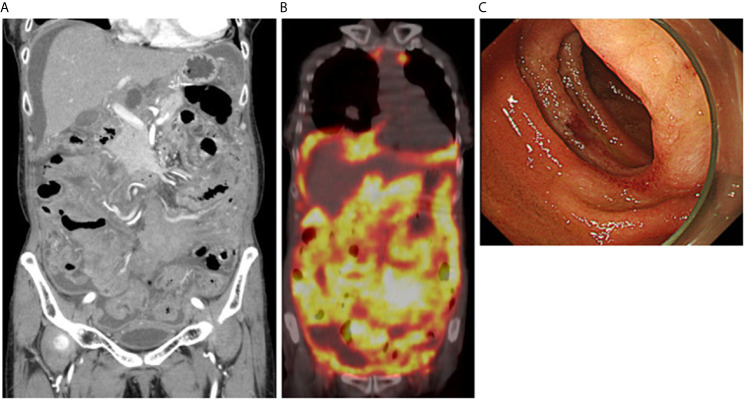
Massive accumulation of 18F-fluorodeoxyglucose into the abdominal cavity in positron emission tomography. (A) Moderate ascites and wall thickening of the jejunum and ileum were seen in contrast-enhanced computed tomography. (B) Massive accumulation of 18F-fluorodeoxyglucose was observed into the peritoneum and small bowel wall. (C) An endoscopic image of a jejunum tumor detected by double balloon-assisted enteroscopy. A whitish elevated tumor was seen in the proximal jejunum.
